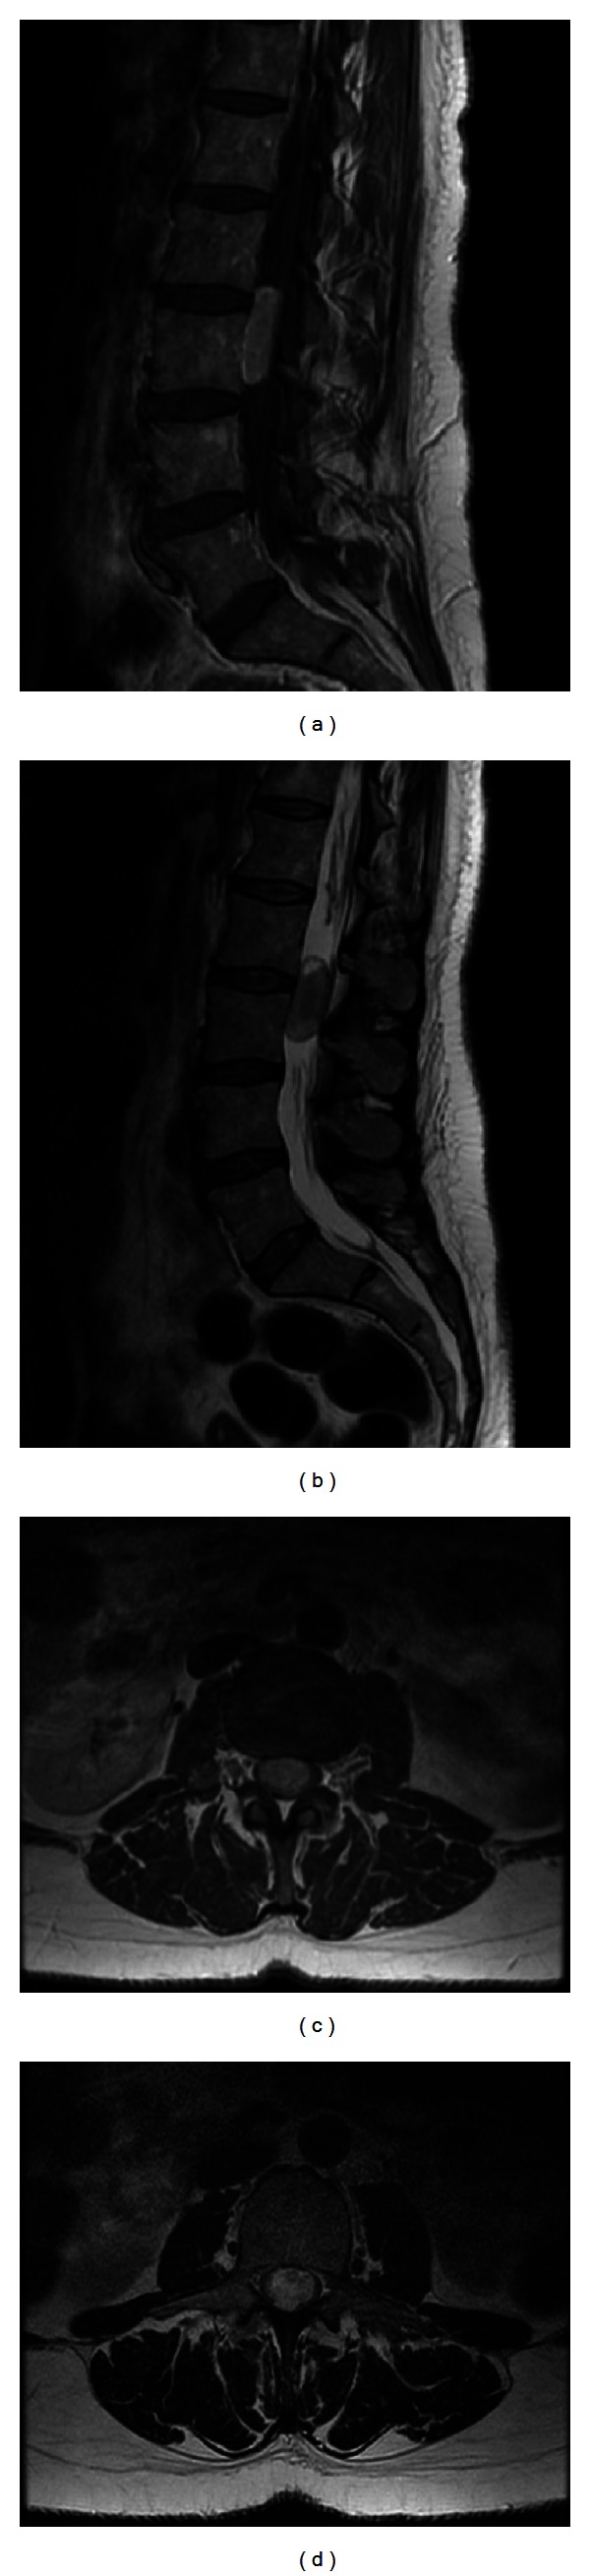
(a) Pre-op sagittal T1 with contrast MRI showing L2-L3 paraganglioma. (b) Pre-op sagittal T2 MRI showing L2-L3 paraganglioma. (c) Pre-op axial T1 with contrast MRI showing L2-L3 paraganglioma. (d) Pre-op axial T2 MRI showing L2-L3 paraganglioma.
